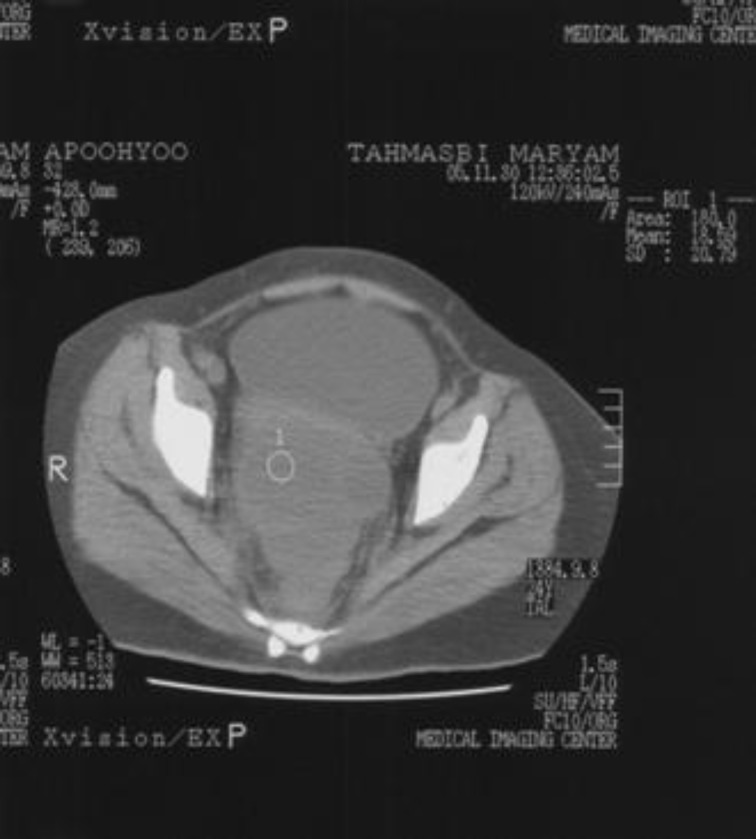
Preoperative CT-Scan demonstrating an ill-defined pelvic mass
